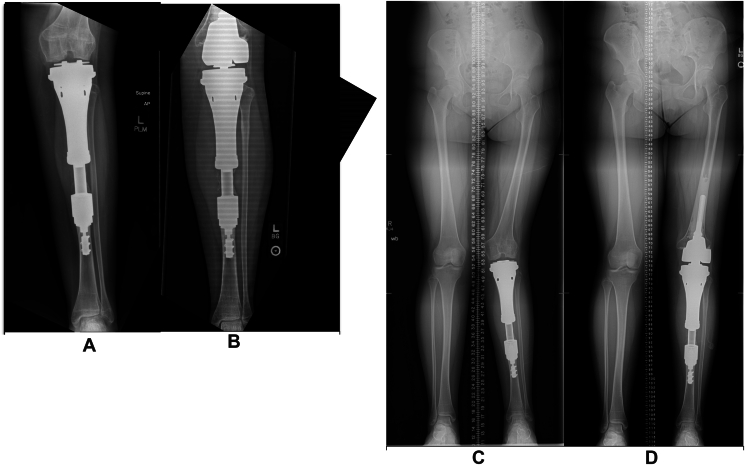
A. Prerevision radiograph showing condyle wear. B. Postrevision radiograph. C. Prerevision scanogram showing knee valgus deformity. D. Postrevision scanogram.
